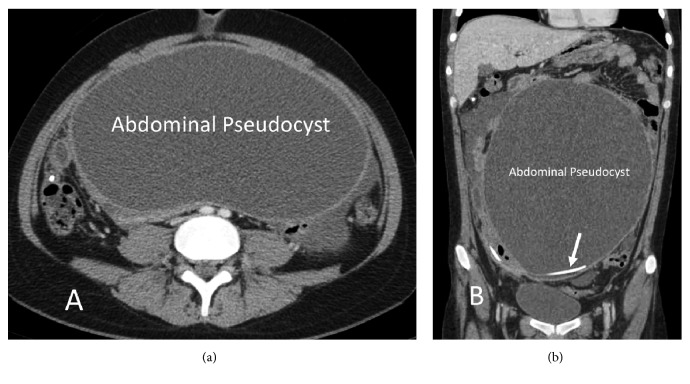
Axial (a) and coronal (b) noncontrast CT reformatted images at about 9 months after ventriculoperitoneal shunt placement. Large thick-walled abdominal pseudocyst with smooth walls. Note VP shunt catheter within the base of the cyst (arrow, b).
